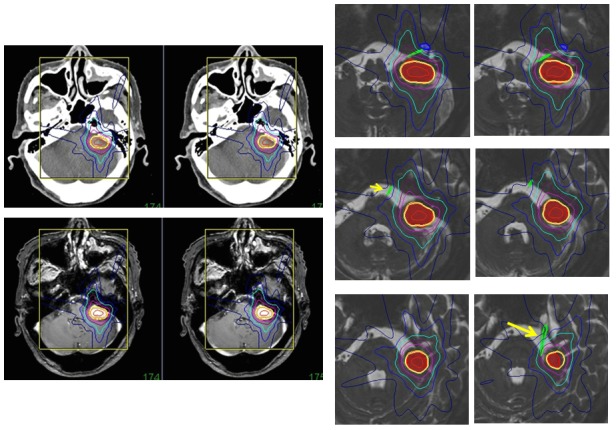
A cerebello-pontine angle tumor and the surrounding structures. A Gd-enhanced MR image (lower left) fused to thin-sliced CT (upper left). The image fusion of a heavy T2-image showing the dose plan sparing the 7th and 8th nerves (green), cochlea (blue), 6th nerve (small yellow arrow), and 5th nerve (large yellow arrow).
